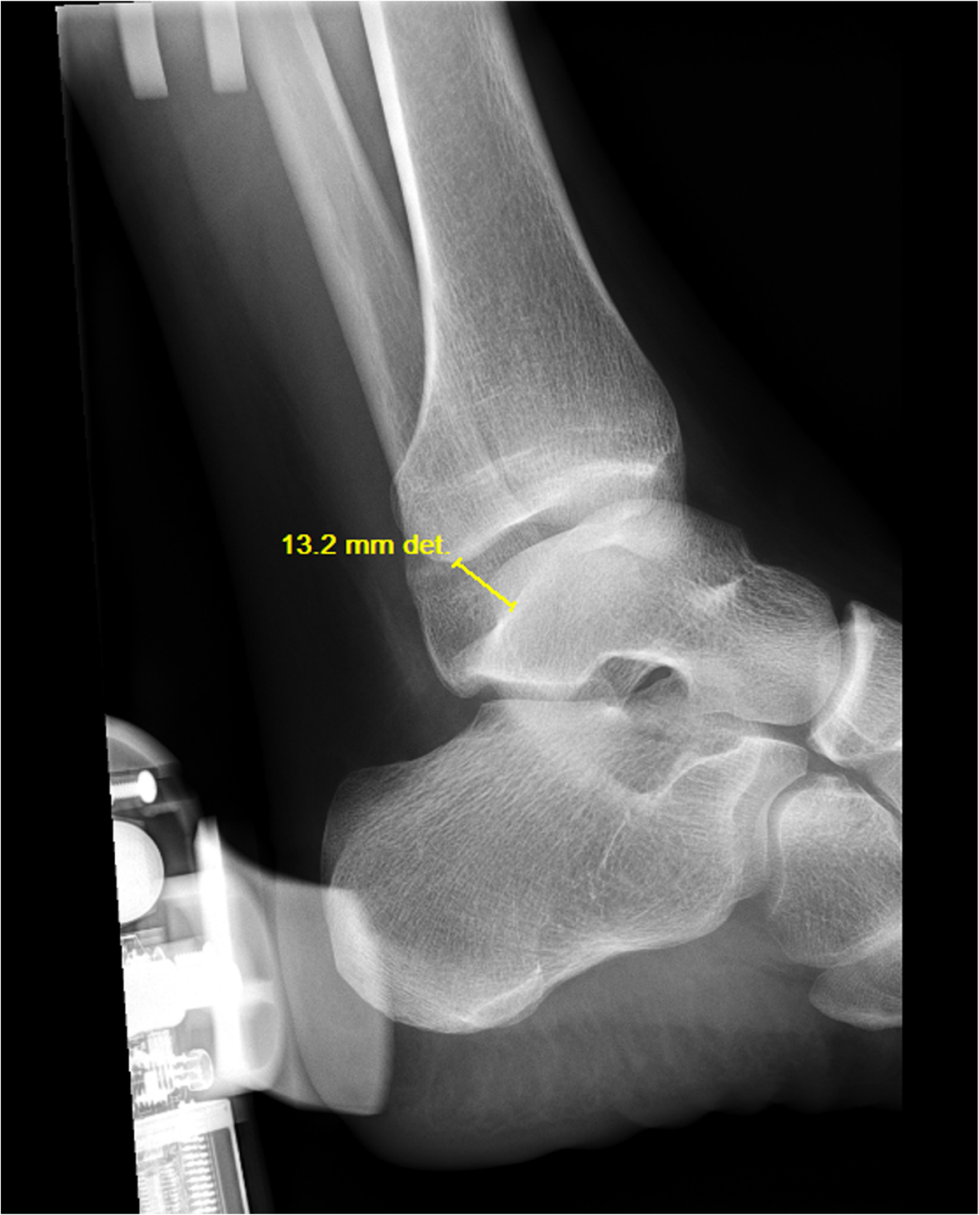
Anterior translation of the talus during the anterior drawer stress test is measured as the distance on X-ray from the posterior lip of the tibial joint surface to the nearest point of the talar dome (left ankle, 13.2 mm in this image)
